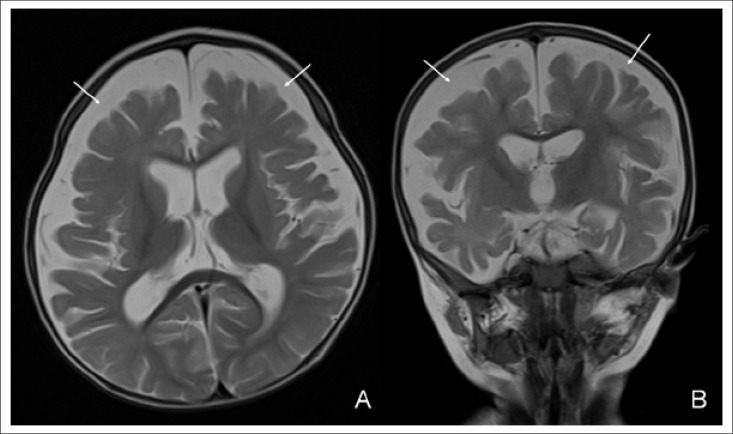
Initial MR images: axial (A) and coronal (B) T2-weighted images showed severe cerebral atrophy with enlargement of cortical sulci and subarachnoid spaces (arrows)
